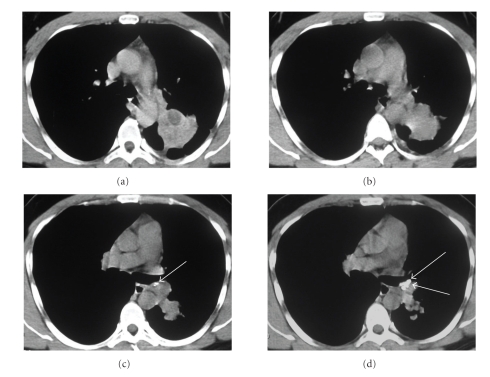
HRCT scans with mediastinal window (a)–(d) demonstrated a mass with irregular borders, measuring about 6 cm in diameter, with pleural extension and thickening of adjacent pleura, located in the upper segment of the left lower lobe. The mass was heterogeneous with hypodense areas. Amorphous calcifications were also present in the mass (c, d) (arrows).
